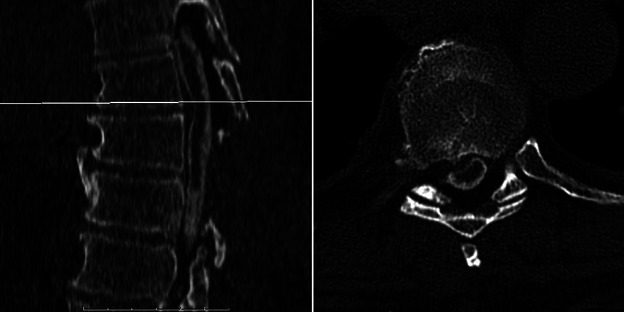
Preoperative focused computed tomographic myelogram, sagittal (left) and corresponding axial (right) views, showing right paracentral subligamentous disk herniation at T7–8 with mass effect on the cord.
